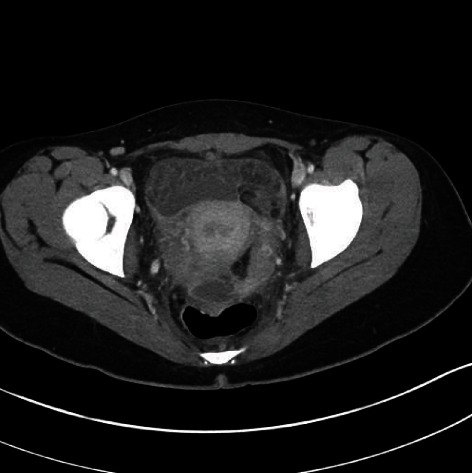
Initial enhanced abdominal CT scan showing free pelvic fluid.
